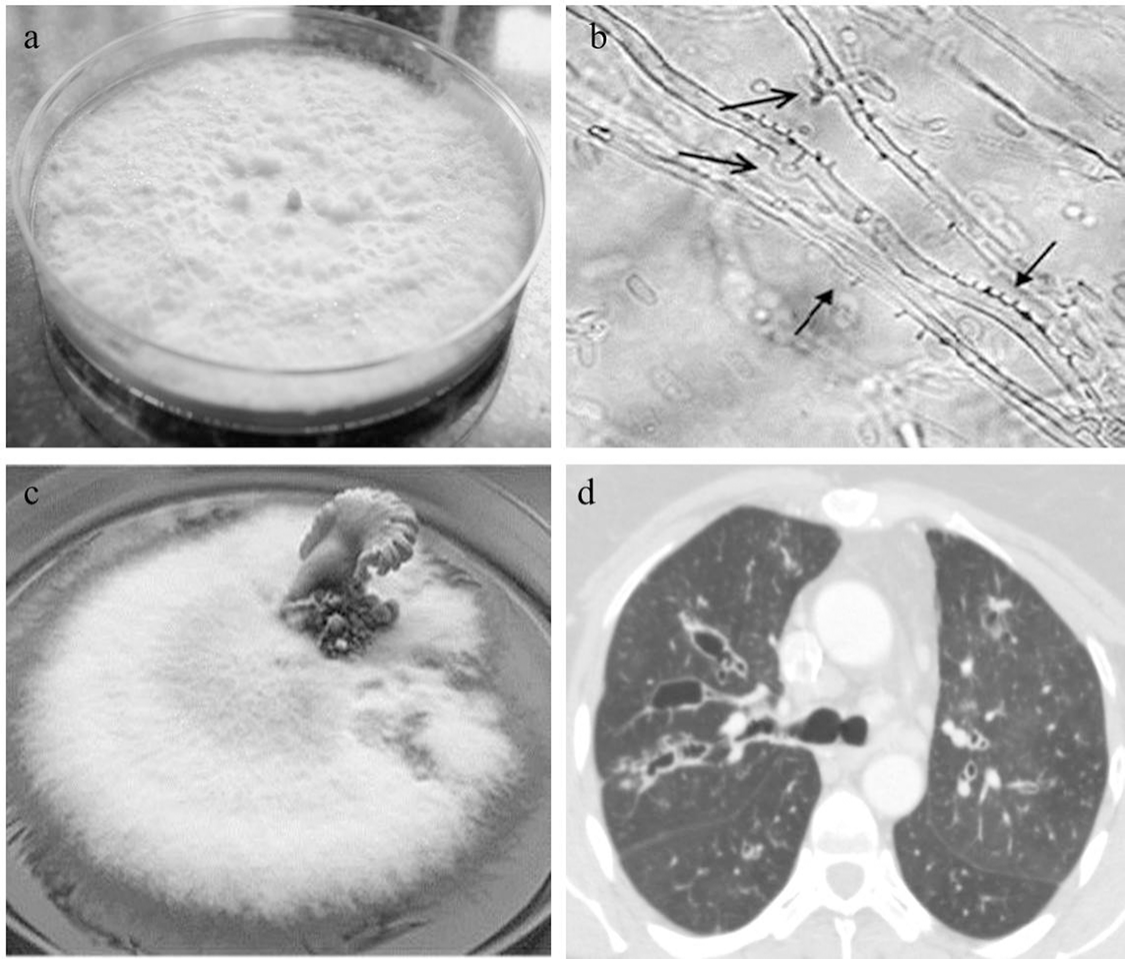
Culture characteristics of *Schizophyllum commune* and computed tomography image of a patient with allergic *S*. *commune* infection. (a) Sabouraud’s dextrose agar petri plate showing white cottony growth of non-sporulating basidiomycete (*Schizophylum commune*) after 10 days of incubation at 28°C. (b) Lactophenol cotton blue mount of slide culture on potato dextrose agar of *S*. *commune* showing clamp connections and spicules after 2 weeks of incubation at 28°C (400x). (c) Plate showing basidiocarp (fruiting body) of *S*. *commune* after 4–5 weeks of incubation at 28°C with periodic exposure to light. (d) High-resolution computed tomography of thorax of patient with allergic bronchopulmonary mycosis due to *S*. *commune* showing central bronchiectasis in both the upper lobes.
